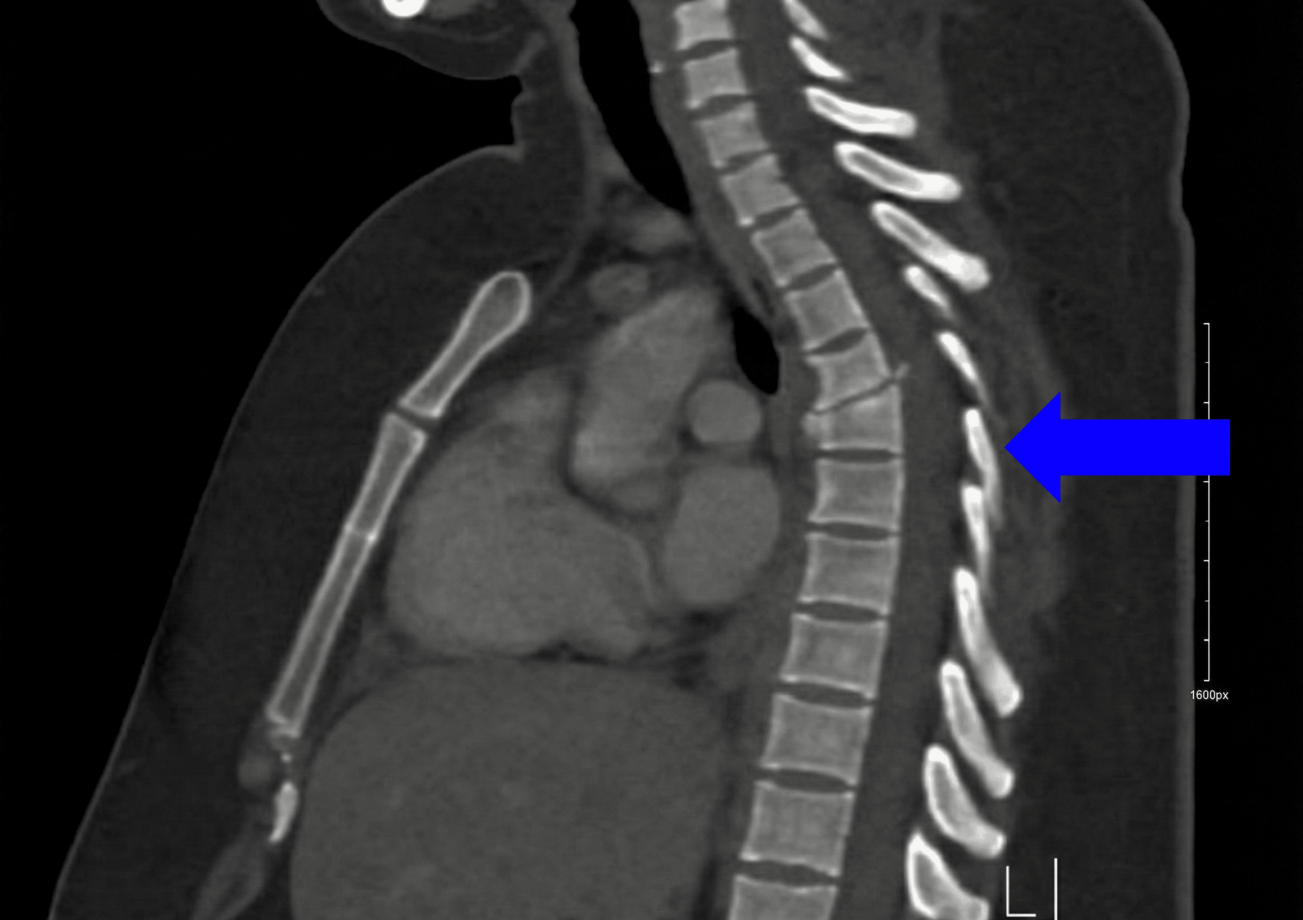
Sagittal CT scan of the thoracic spine Sagittal CT scan showing the congenital absence of the spinous processes.
